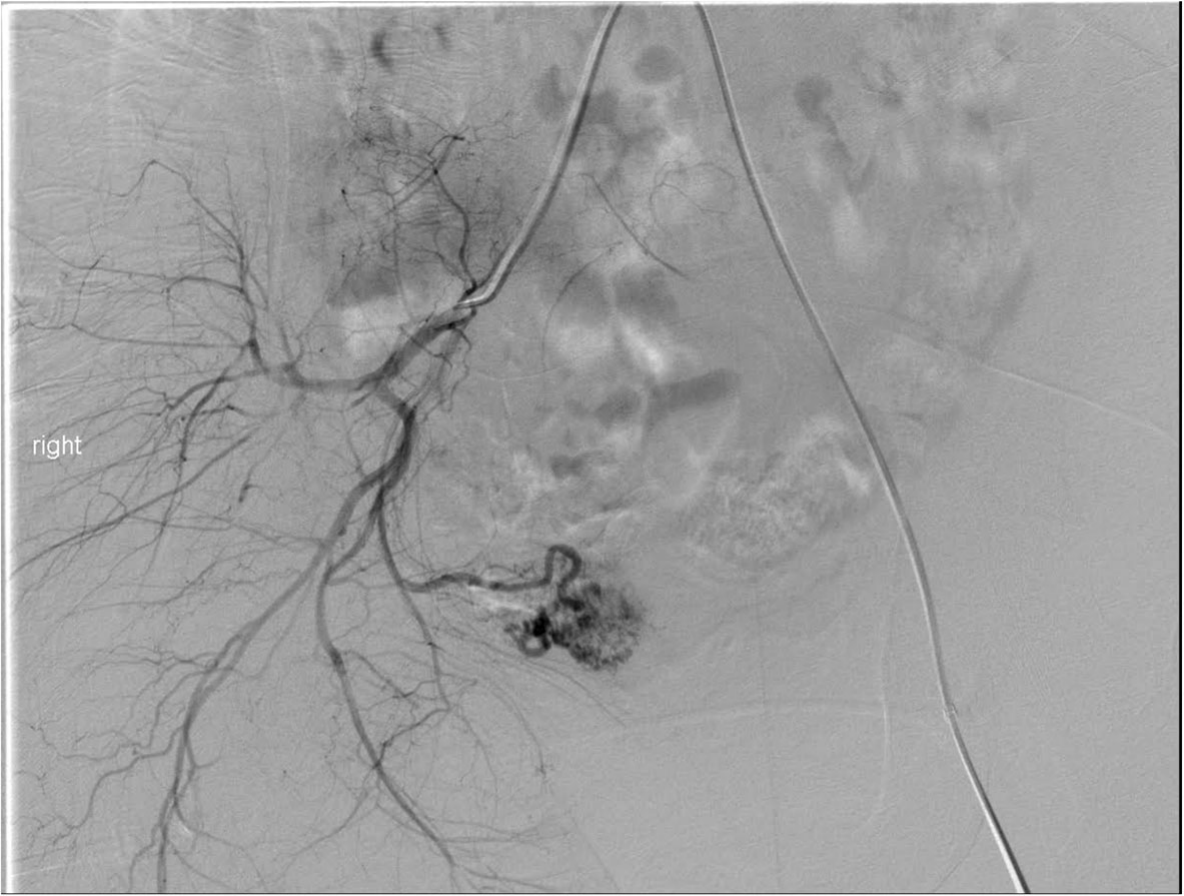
Selective angiography of the right common iliac artery demonstrates an arteriovenous malformation that corresponds to the flow voids seen on the MRI in Fig. 6
